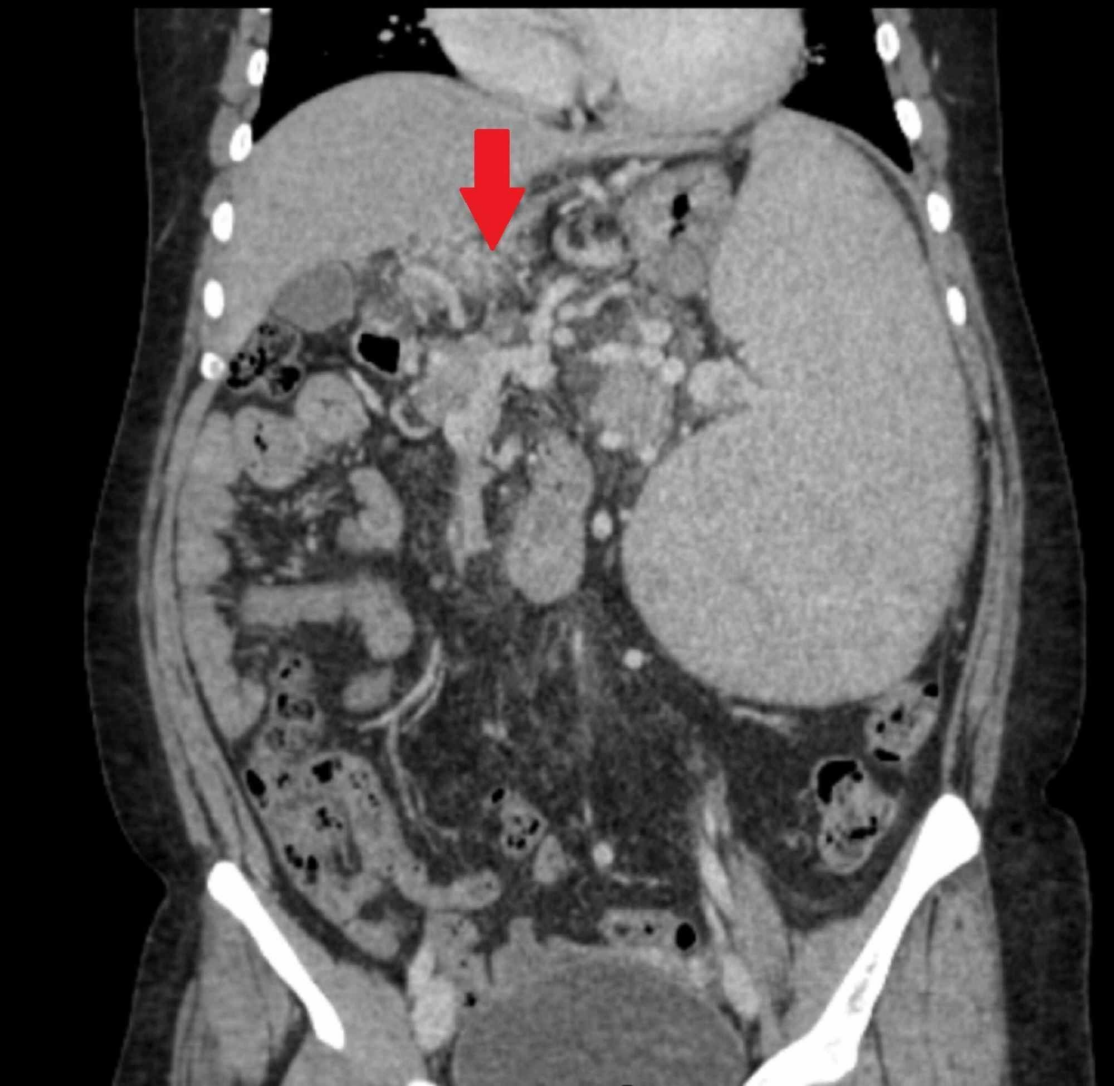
CT scan of abdomen (coronal view) showing collaterals or cavernoma formation around PV CT, computed tomography; PV, portal vein
